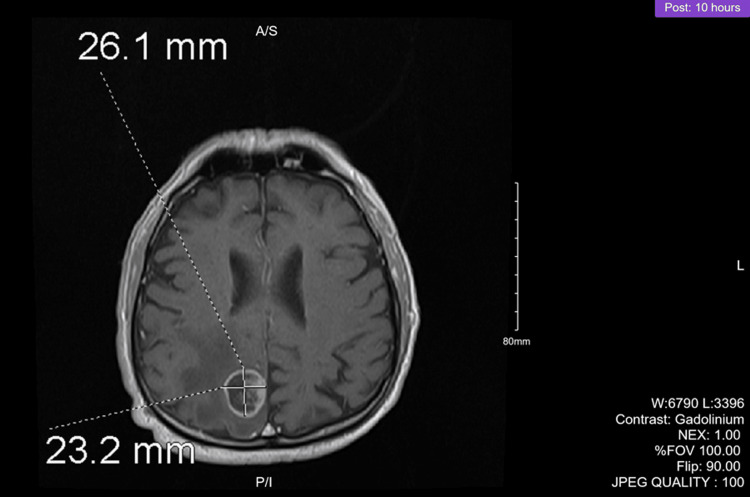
Preoperative brain MRI coronal view MRI: magnetic resonance imaging
